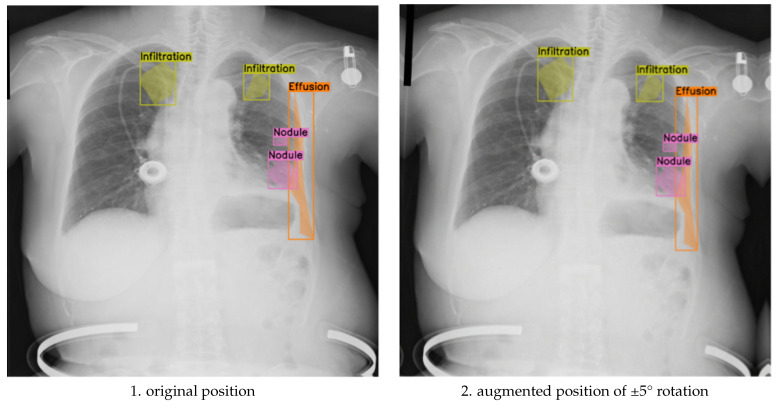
Comparison of original and discrete rotations at (−5°, +5°) augmented chest X-ray images.
